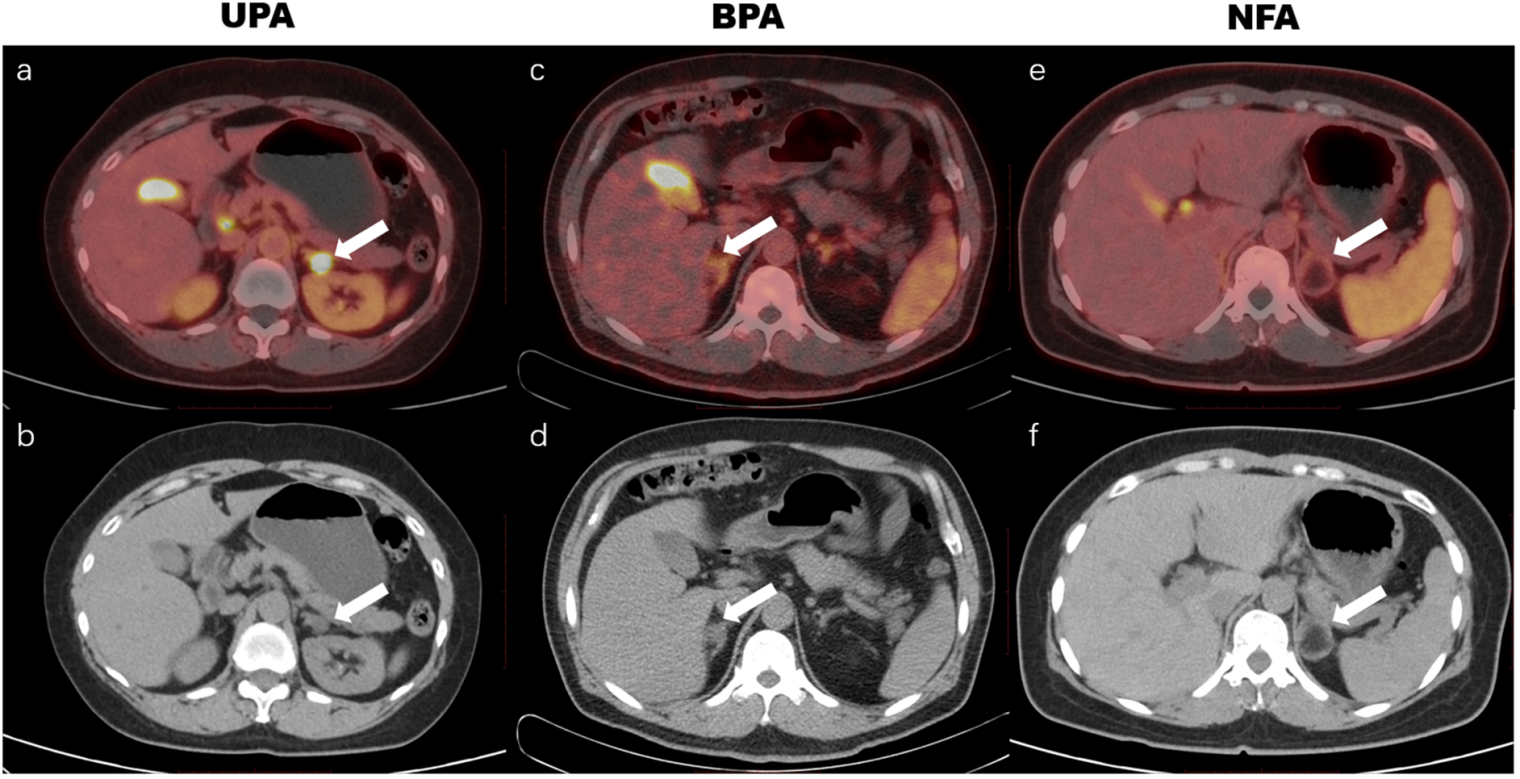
^18^F-AlF-NOTA-pentixafor PET/CT imaging of patients with unilateral primary aldosteronism (UPA), bilateral primary aldosteronism (BPA), and nonfunctional adenoma (NFA). The UPA patient was a 61-year-old woman with hypertension for 10 years and hypokalemia for 2 years, with maximum blood pressure of 163/105 mm Hg, and minimum blood potassium of 2.69 mmol/L. A positive uptake (SUV_max_ of 31.5, white arrow in a, b) was observed in the left adrenal nodule, and postoperative pathology revealed a left adrenal cortical adenoma. The BPA patient was a 74-year-old man with hypertension for 13 years and hypokalemia, maximum blood pressure of 160/99 mm Hg, and minimum blood potassium of 3.05 mmol/L. ^18^F-AlF-NOTA-pentixafor PET/CT showed a warm lesion in the right adrenal (white arrow in c, d) with an SUV_max_ of 4.0. The lateralization index (LI) based on adrenal venous sampling (AVS) was 1.3, which indicated bilateral lesions. The NFA patient was a 46-year-old woman with an incidental adrenal nodule and aldosterone/renin ratio of 138.5, without hypertension or hypokalemia. The ^18^F-AlF-NOTA-pentixafor PET/CT exhibited a cold lesion in the left adrenal (white arrow in e, f) with an SUV_max_ of 2.9, and postoperative pathology revealed a left adrenal medullary lipoma.
